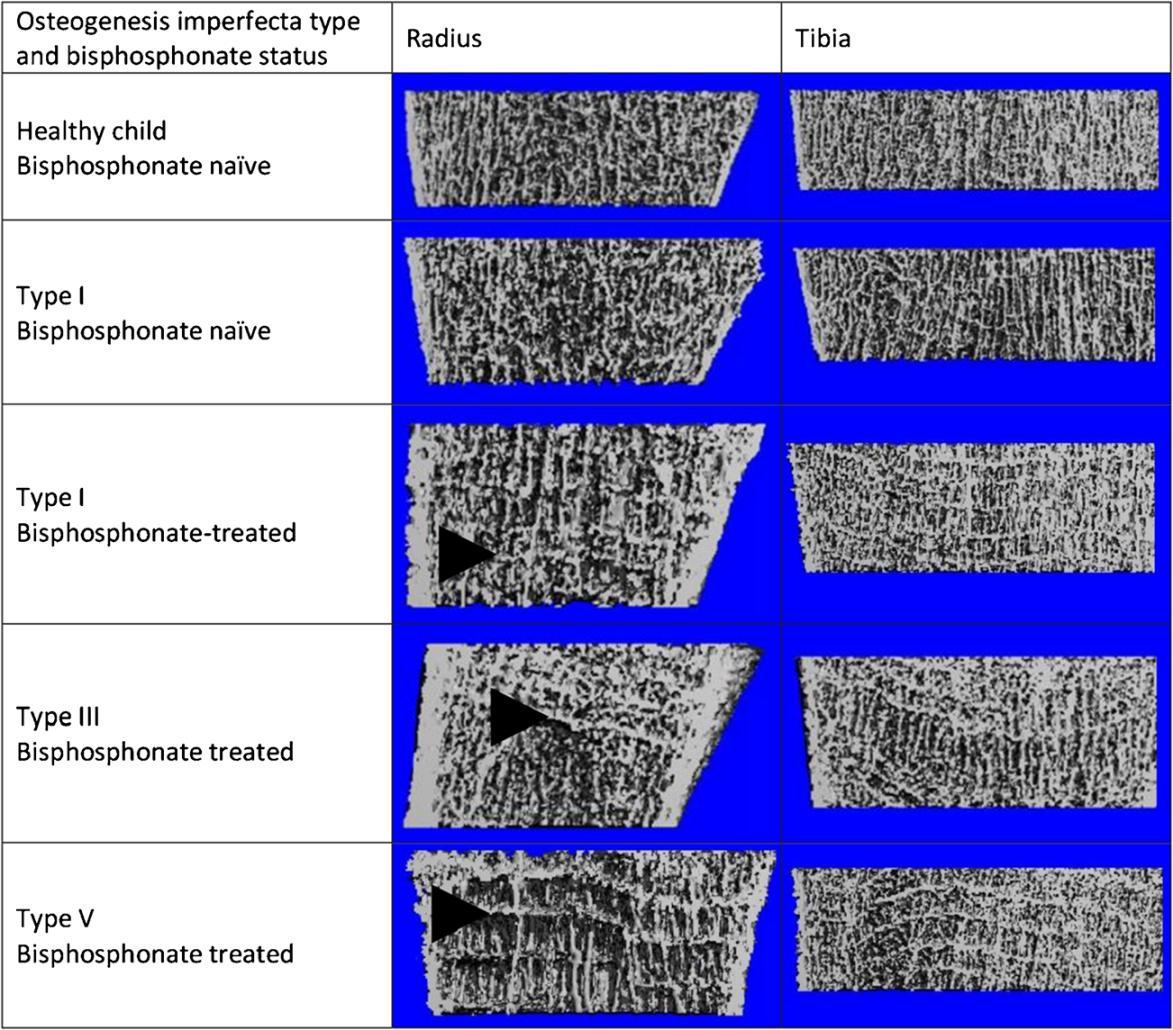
Coronal slices of non-dominant radii and non-dominant tibiae produced by high-resolution peripheral quantitative CT scans of children with osteogenesis imperfecta. Note the high resolution of the images and the horizontal bisphosphonate, or “zebra”, lines in bisphosphonate-treated children (*arrowheads*), which should not be mistaken for trabeculae. The bisphosphonate lines appear more pronounced in the radius, possibly because of image magnification, although increased metabolic activity at the wrist compared to the ankle might play a role
